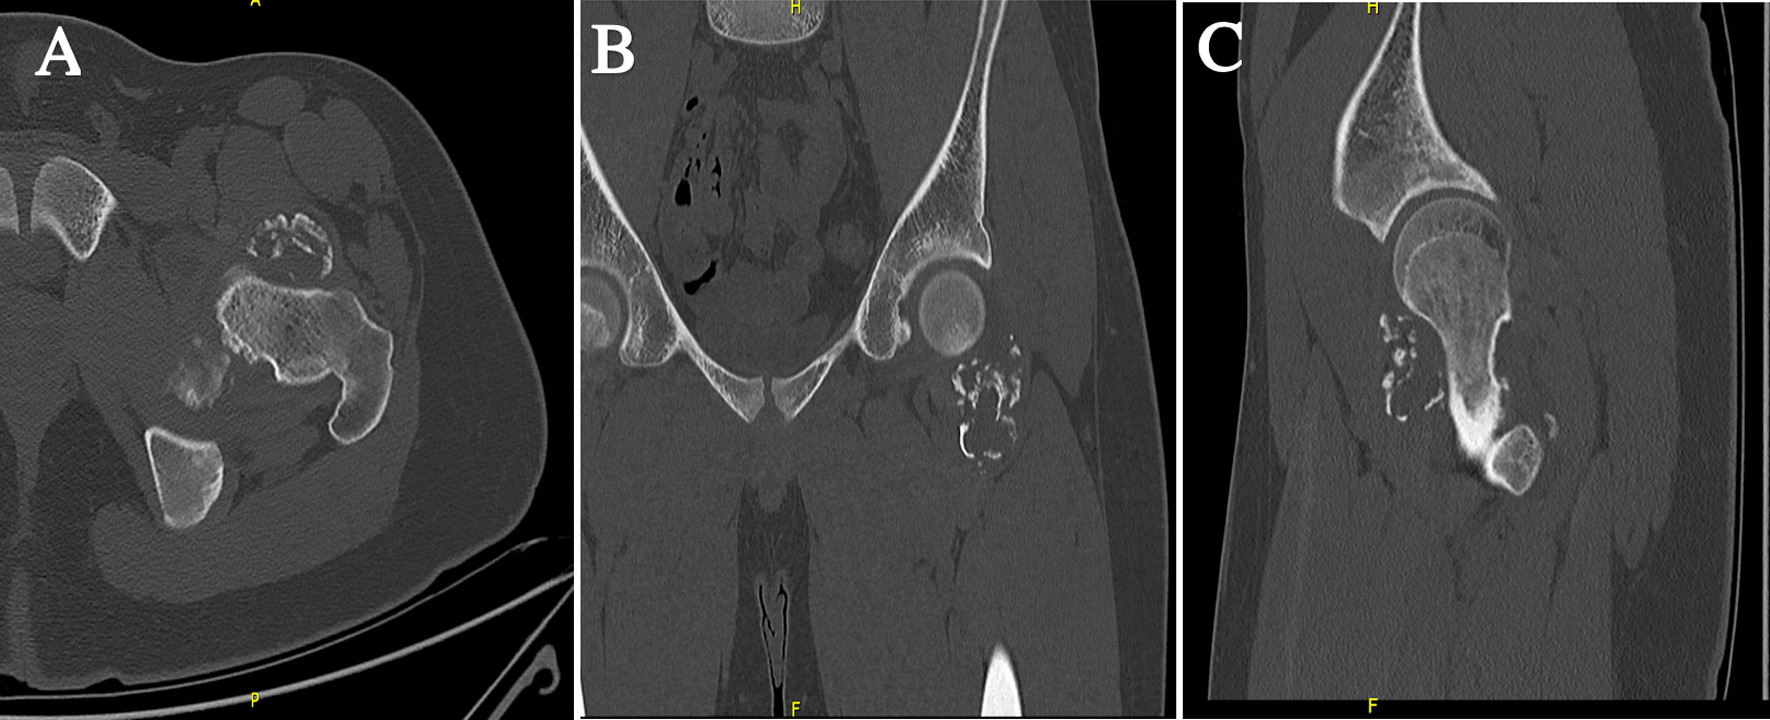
A Axial, B coronal, and C sagittal CT scans of the left hip showing the anterolateral lesion with a cartilage cap
